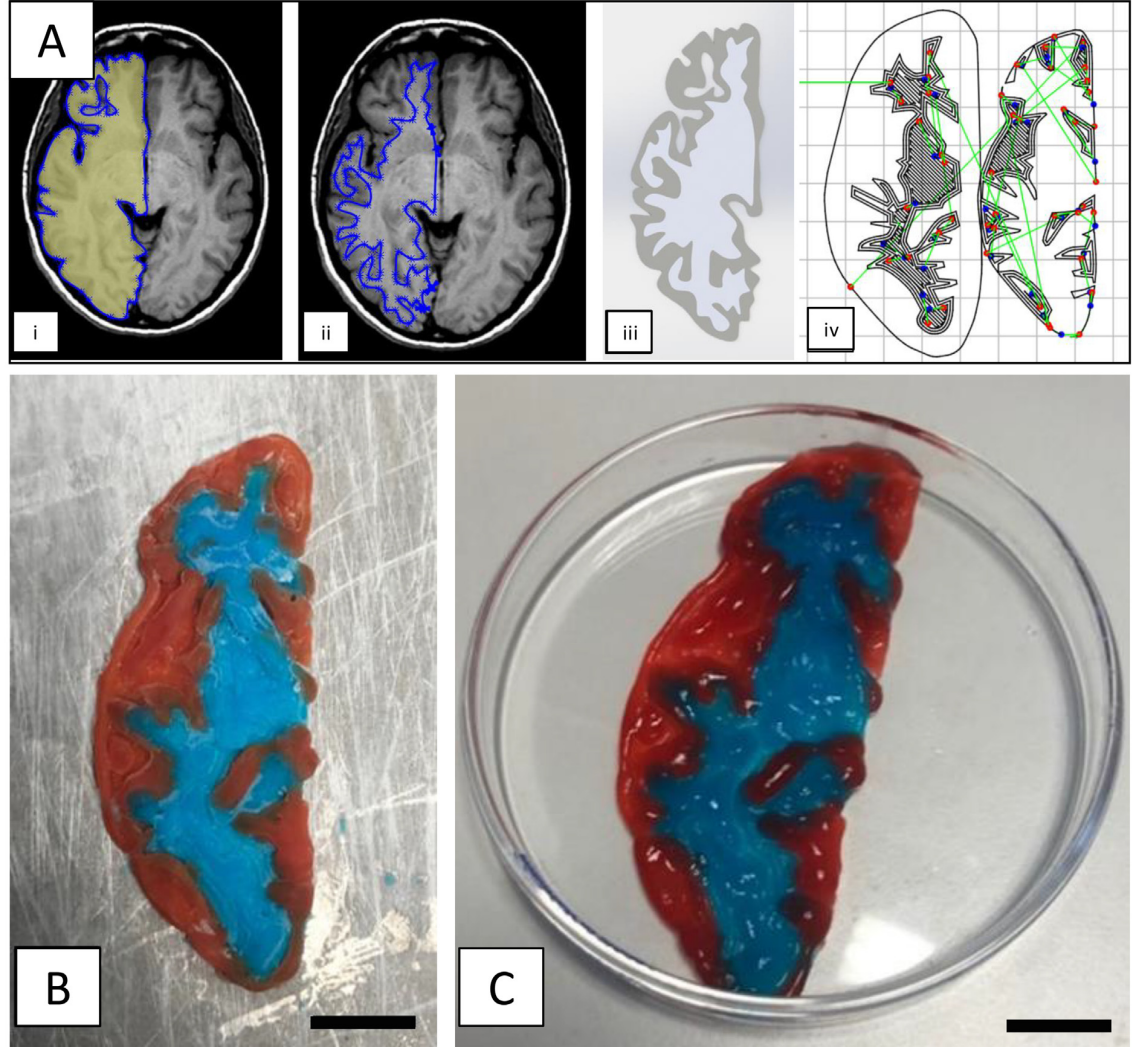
(A) Preparation of 3D printed brain slice from MRI. (i) outlined bitmap trace of the left hemisphere of a human brain MRI [31]; (ii) outlined bitmap trace of white matter areas in the left hemisphere; (iii) output STLs of white and grey matter regions and (iv) extruder path obtained using Slic3r and MATLAB post-processing, and (B) 3D printed brain slice with detailed grey and white matter geometry represented in red and blue, respectively, still frozen just after print completion and (C) final 3D printed brain slice thawed in petri dish after removal from 3D printing platform. Scale bar: (B-C) 10 mm. (For interpretation of the references to colour in this figure legend, the reader is referred to the web version of this article.)
